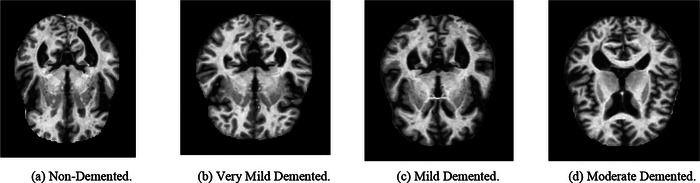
MRI of different Alzheimer's disease classes.
